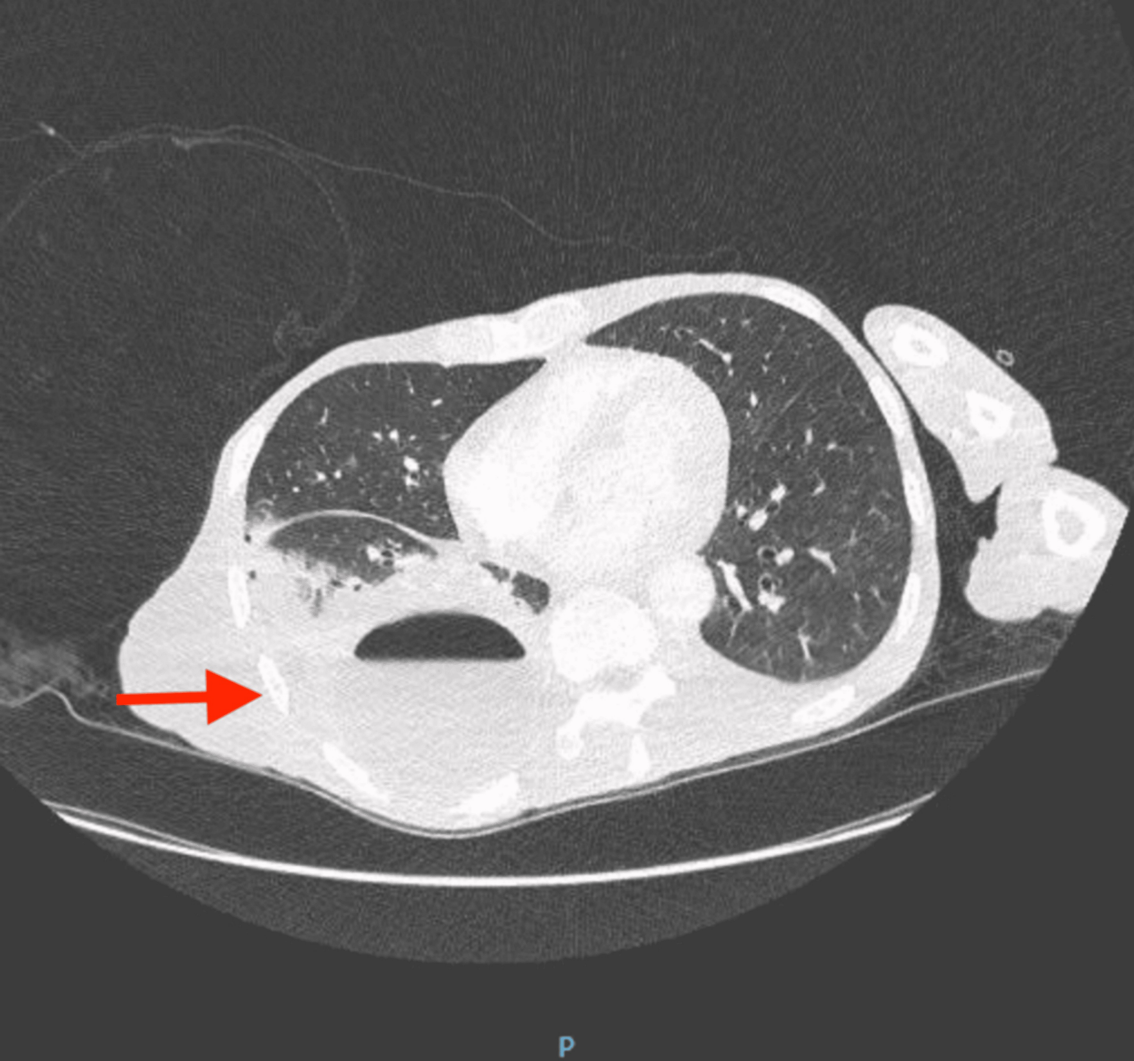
CT chest showing multiple loculated fluid collections in the right lower lobe.
